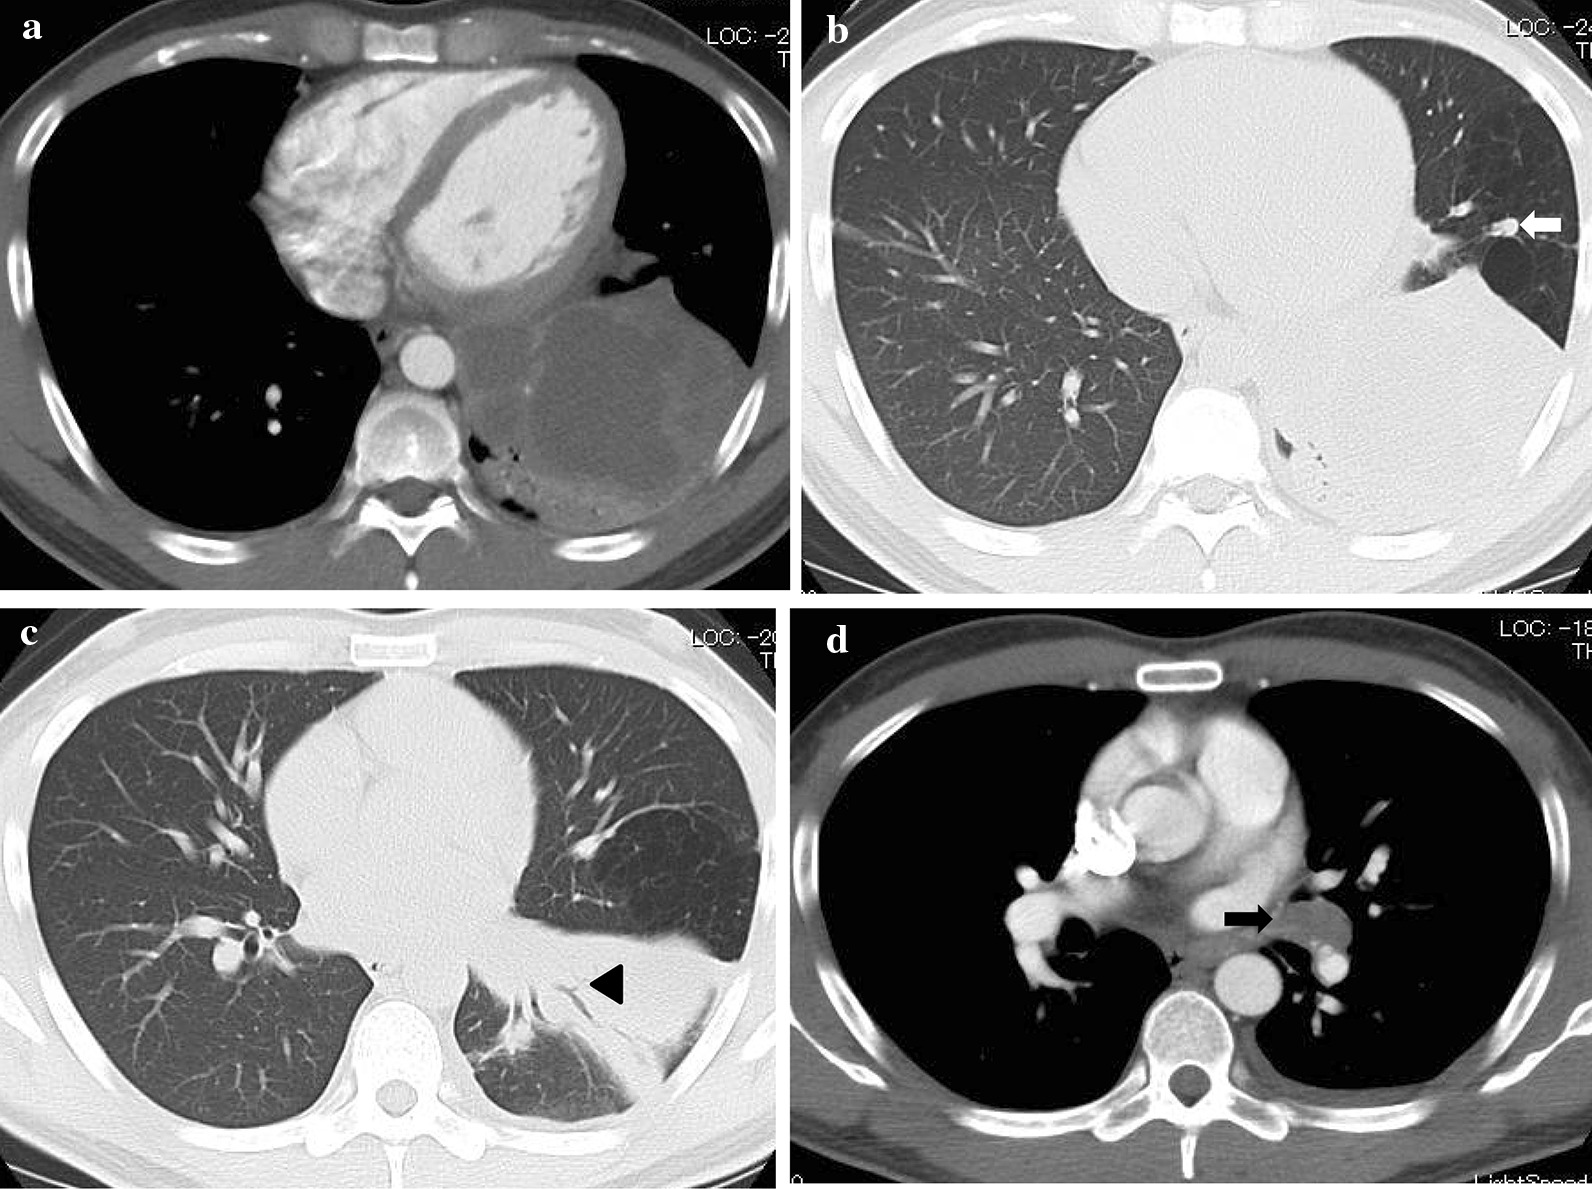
Contrast-enhanced CT performed on admission revealing an abscess, emphysematous changes, ectatic bronchi, a nodular lesion that was considered a mucoid impaction of the lingular segment (arrow), and disrupted bronchus within the left lower lobe (arrowhead) (a–c). A small mass lesion near the left second carina (arrow) is also shown (d)
